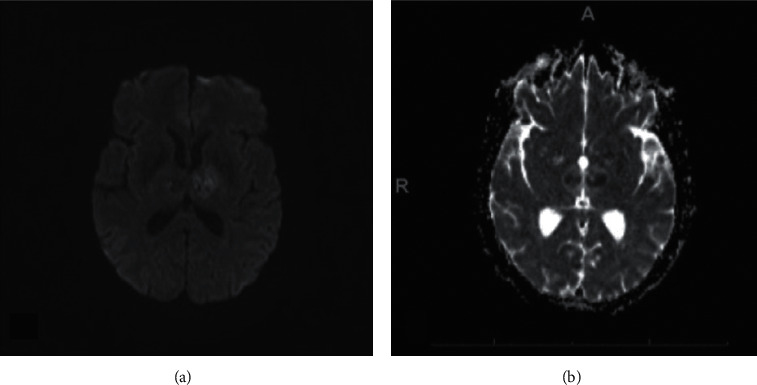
Axial DWI (a) and axial ADC (b) demonstrate subacute ischemic infarct bilaterally in the thalamus with restricted diffusion and low ADC values.
